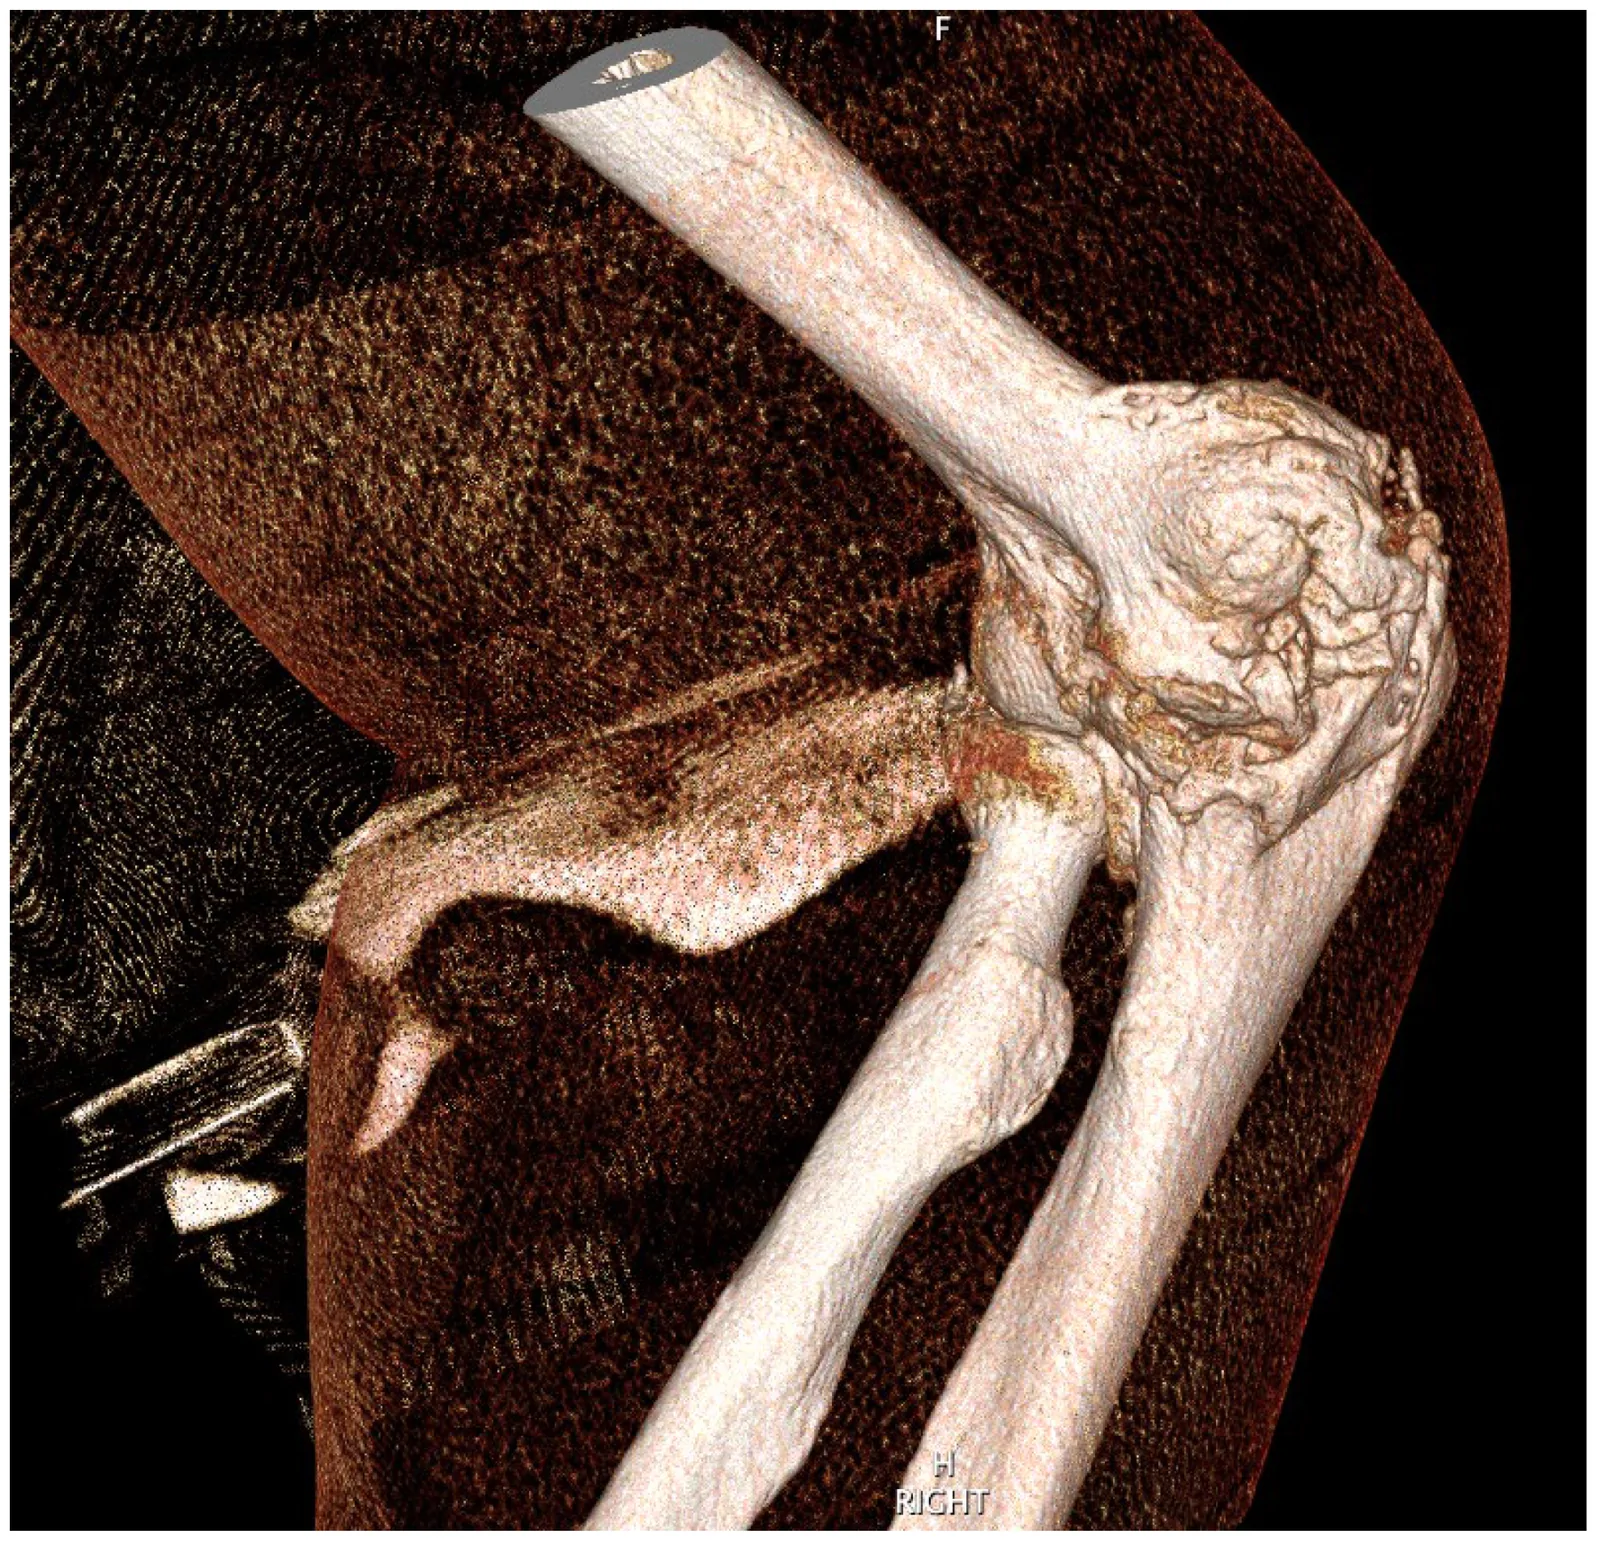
Preoperative 3D computed tomography rendering demonstrating severe heterotopic ossification with near complete bridging across the ulnar collateral ligament, lateral ulnar collateral ligament, and posteromedial aspect of the elbow. This image was used preoperatively to guide treatment.
